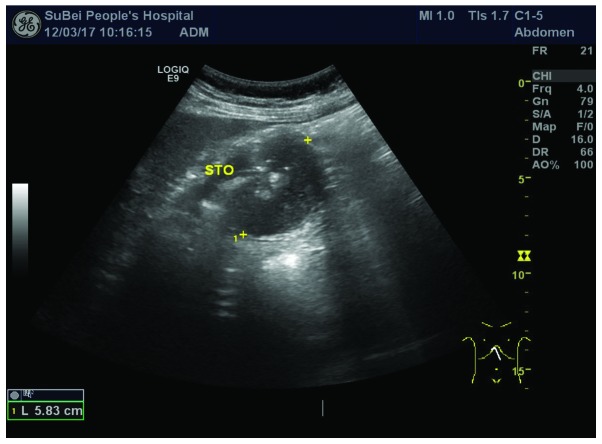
Pre-operative abdominal ultrasound revealing a mass in the posterior wall of the stomach.
